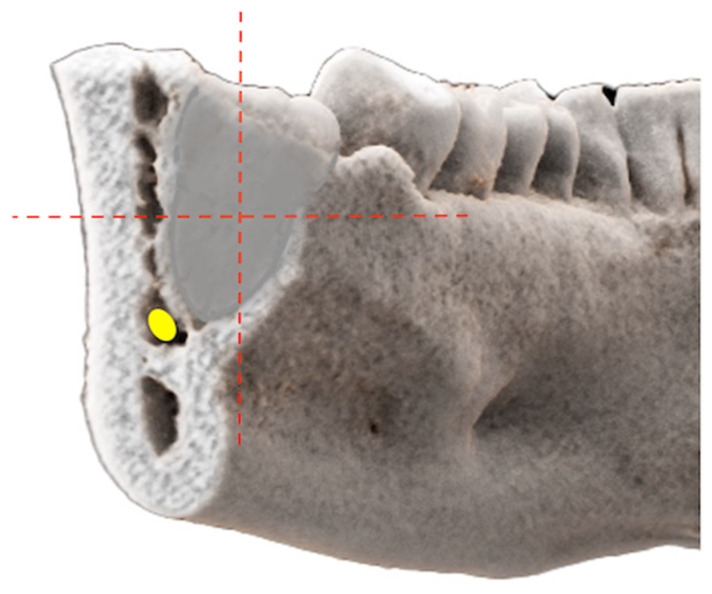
Photorealistic three-dimensional (3D) visualization of a study participant’s cone-beam computed tomography (CBCT) using cinematic rendering (CR) image reconstructions. In the coronal reference layer, showing the closest positional relationship, both readers independently classified the relative positional relationship of the mandibular third molar’s (MTM) roots and the mandibular canal (MC)/inferior alveolar nerve (IAN) by using the Cartesian coordinate system, according to Wang et al. [24] After evaluating whether there was contact or not, the structural center of the MTM was set as the origin in the Cartesian coordinate system and the relative positional relationship—whether the IAN’s position was periradicular (lingual, buccal, inferior) or interradicular (between the roots)—was determined.
